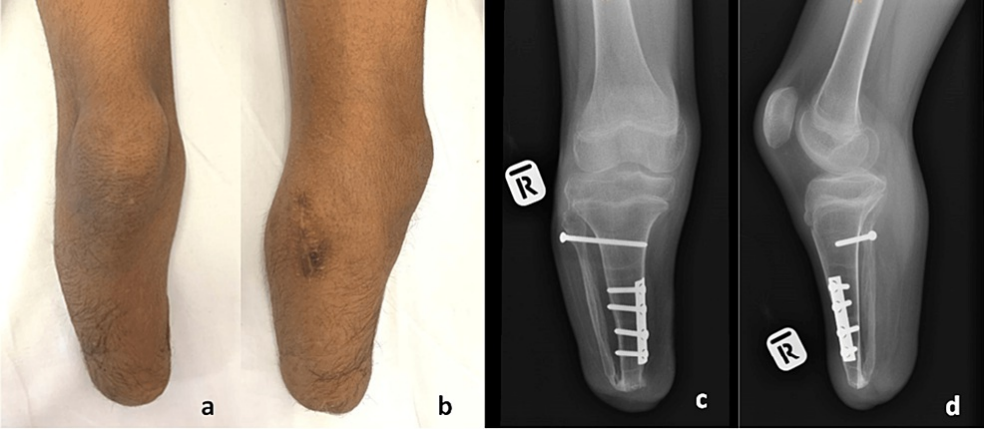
(a,b) Clinical pictures and (c,d) plain radiograph of the patient six months after the surgery. The recurrence of the distal tibiofibular synostosis was observed.
